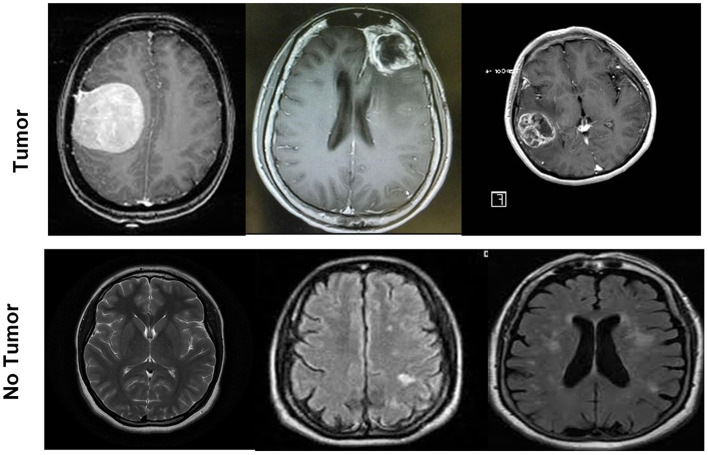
MRI brain images samples for two classes tumor and no tumor.
